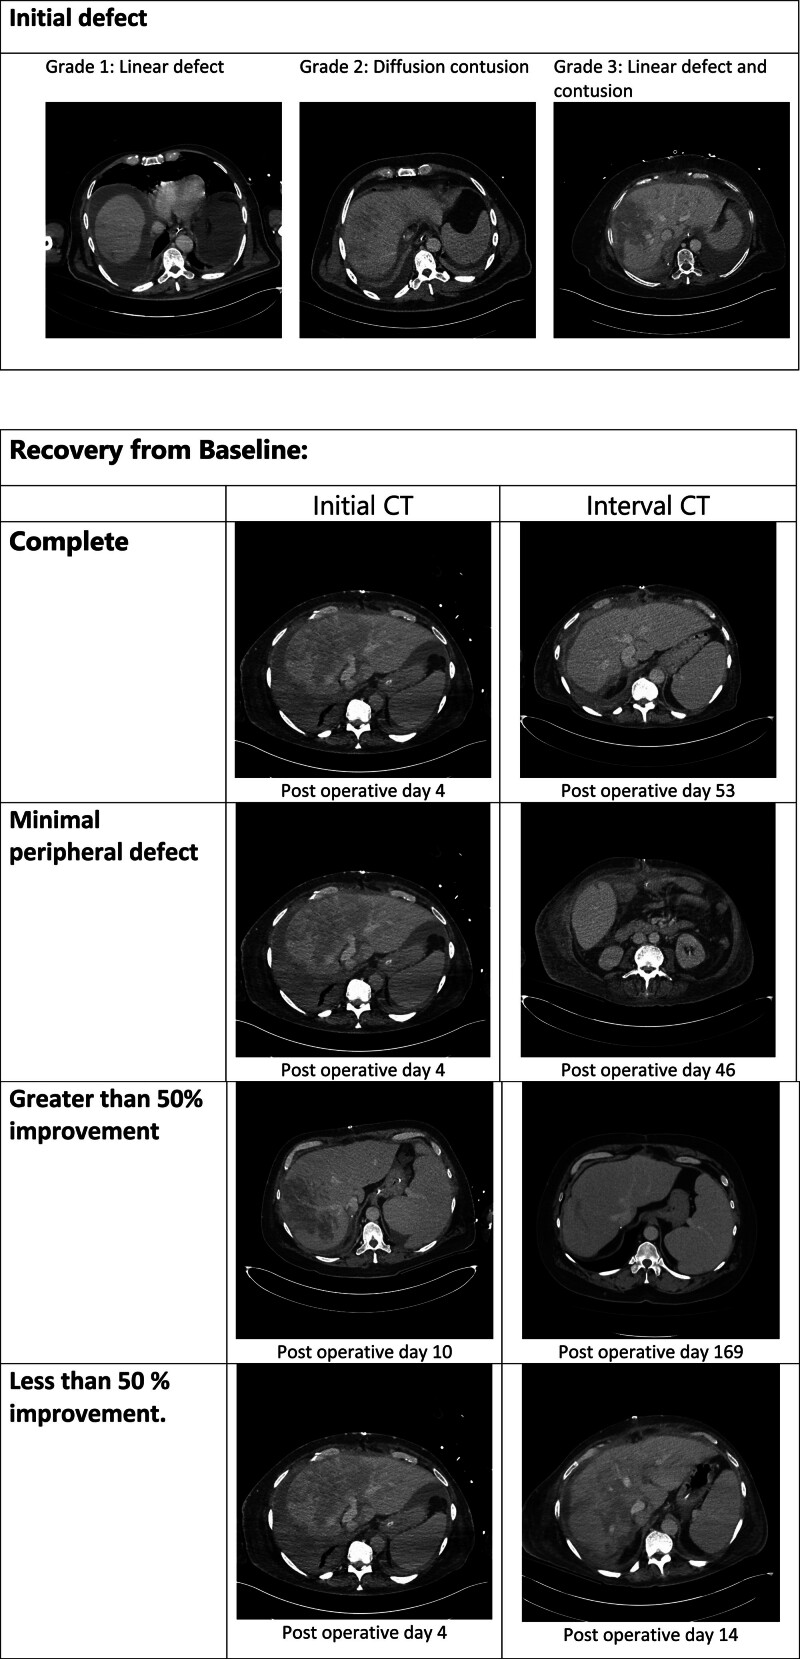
3C classification of cradle compression. Representative images demonstrating the radiological appearances used in the 3C classification of cradle compression. Patients did not undergo protocol CTs during the study period. The recovery from baseline images are from different patients. The CT scans demonstrating <50% improvement, a linear defect and complete resolution are taken from a single patient who had multiple interval postoperative scans. The >50% improvement is from a different patient. 3C, Cambridge Cradle Compression; CT, computed tomography.
